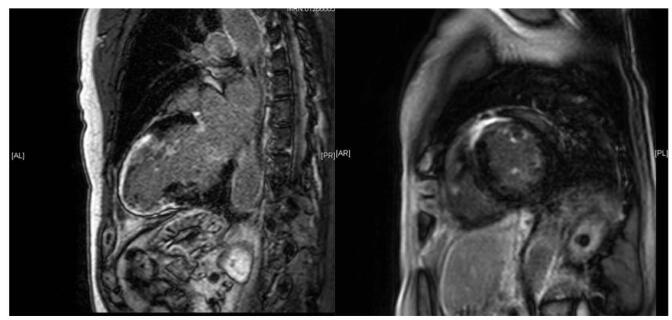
Cardiac MRI (Patient#1): Extensive near transmural LGE in the basal to mid anterior segments extending into all apical segments and subendocardial decreased perfusion at rest, along mid-septal linear mid LGE.
